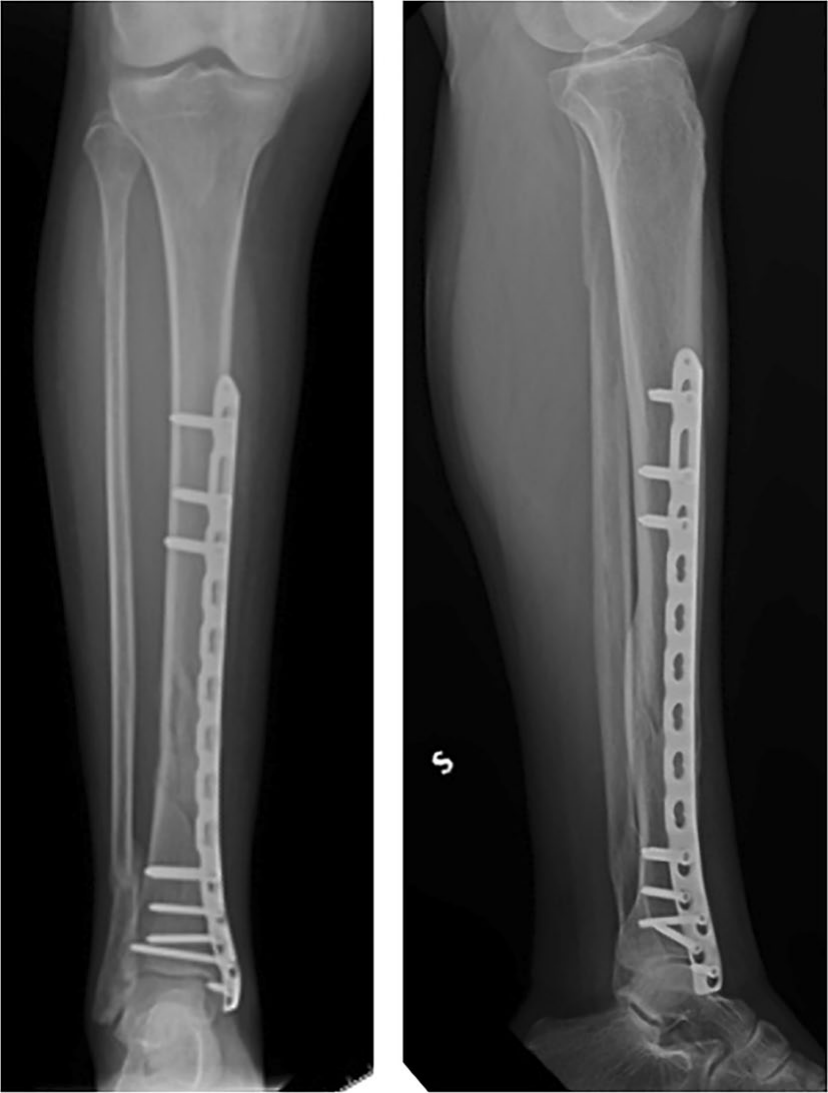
Three months post-operative radiographs show signs of early callus formation with a mRUST score of 10. The fracture went on to heal uneventfully
